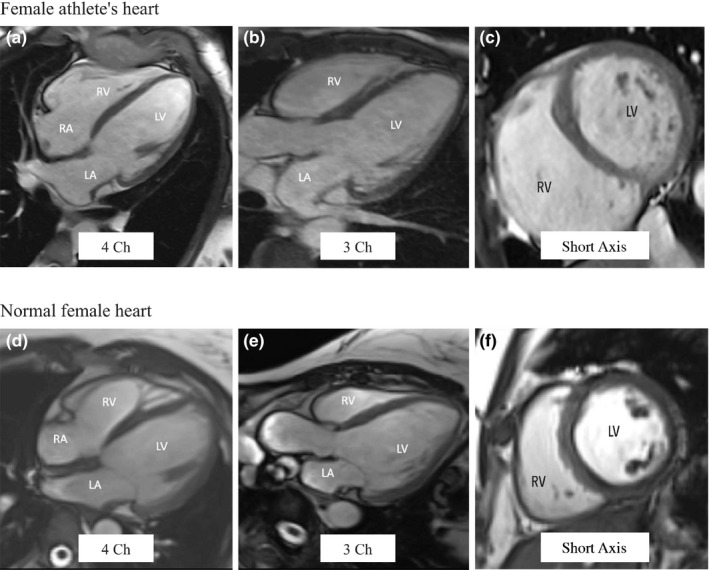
CMR images obtained from an elite female athlete and a normal healthy female. Images obtained from an elite female athlete include four chamber (a), three chamber (b), and short axis (c) views. Measurements obtained are as follows: LVEDV 102 ml/m^2^, LVESV 39 ml/m^2^, LVSV 63 ml/m^2^, and LVEF 62%, RVEDV 116 ml/m^2^, RVESV 47 ml/m^2^, RVSV 69 ml/m^2^, RVEF 59%. A CMR from a healthy female is used for visual comparison which include four chamber (d), three chamber (e), and short axis (f) views. Measurements obtained are as follows: LVEDV 80 ml/m^2^, LVESV 35 ml/m^2^, LVSV 46 ml/m^2^, and LVEF 57%, RVEDV 67 ml/m^2^, RVESV 26 ml/m^2^, RVSV 41 ml/m^2^, RVEF 59%. All values not represented with a % are indexed to BSA. This figure highlights differences in ventricular size between female athletes and healthy controls. 2 Ch =2 chamber; 3 Ch =3 chamber; 4 Ch =4 chamber. BSA, body surface area; CMR, cardiac magnetic resonance imaging; LV, left ventricle; LVEDV, left ventricular end‐diastolic volume; LVESV, left ventricular end‐systolic volume; LVEF, left ventricular ejection fraction; LVSV, left ventricular stroke volume; RV = right ventricle; RVEDV, right ventricular end‐diastolic volume; RVESV, right ventricular end‐systolic volume; RVEF, right ventricular ejection fraction; RVSV, right ventricular stroke volume
